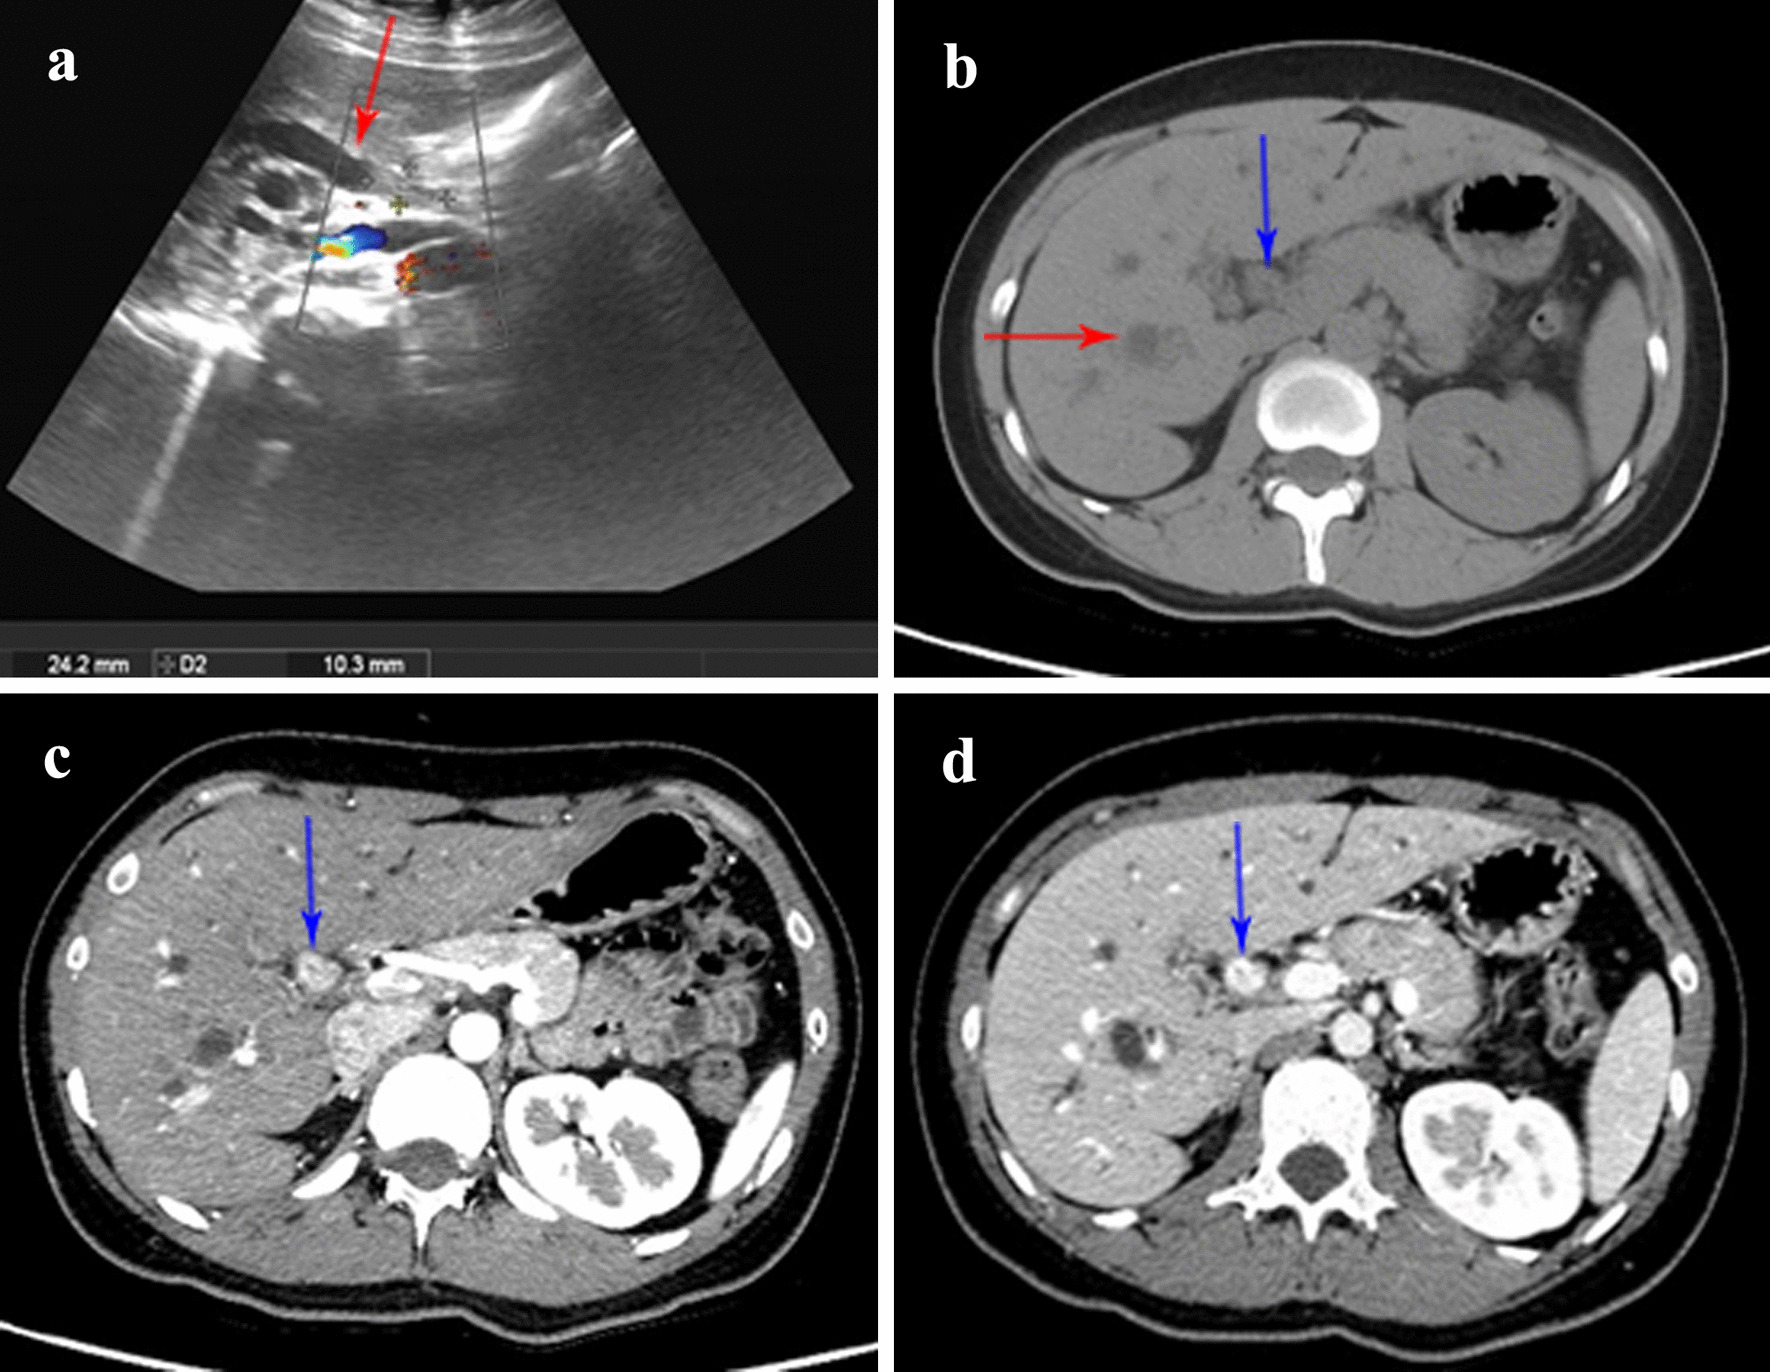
Preoperative abdominal ultrasonography and CT. a Abdominal ultrasonography indicated the dilation of bile duct (red arrow). b Non-enhanced phase of CT showed that the intrahepatic bile duct was marked dilated (red arrow) and the tumor was located in the common hepatic duct (blue arrow). c Arterial-phase of CT showed the tumor was of higher density than liver parenchyma (blue arrow). d Portal-venous phase of CT showed the tumor was of higher density was of higher density than liver parenchyma (blue arrow)
